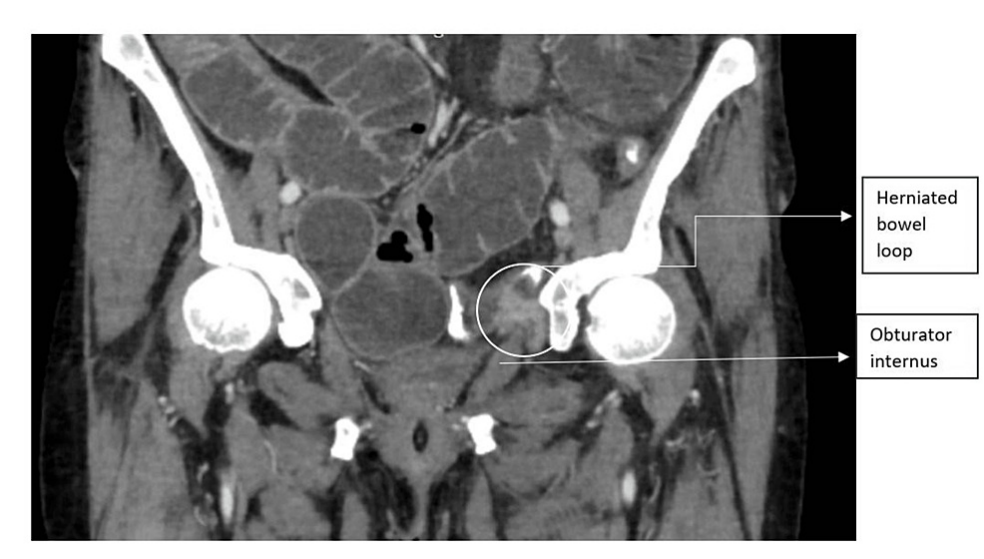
A CECT of the abdomen (coronal view) shows an obturator hernia with dilated small bowel loops. CECT: contrast-enhanced computed tomography
